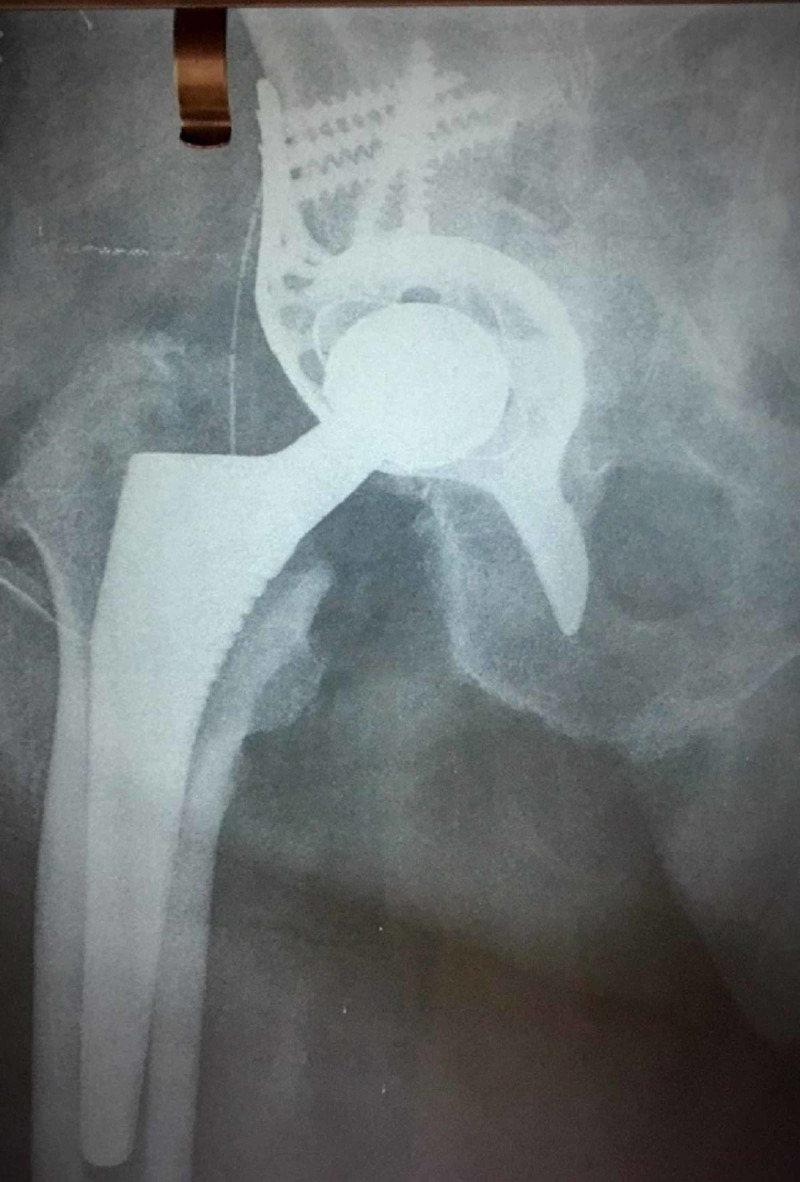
Radiograph of the same patient one year postoperatively
